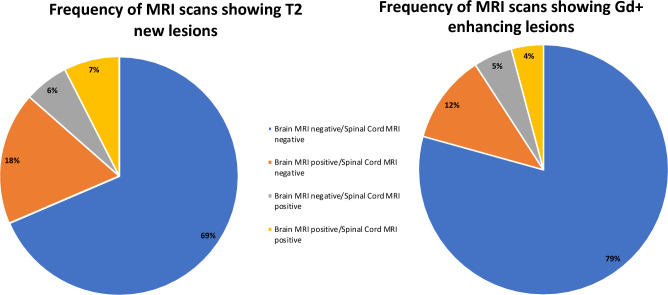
Frequency of MRI scans according to each pattern
